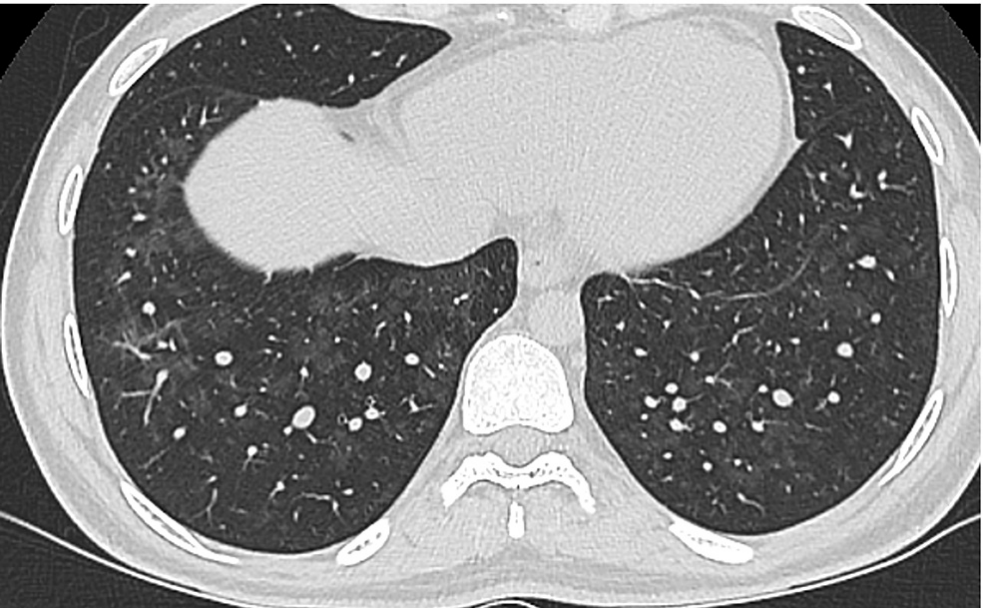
High-resolution computed tomography (HRCT) of the lungs showing complete resolution of bilateral extensive lung nodules two weeks following induction.
